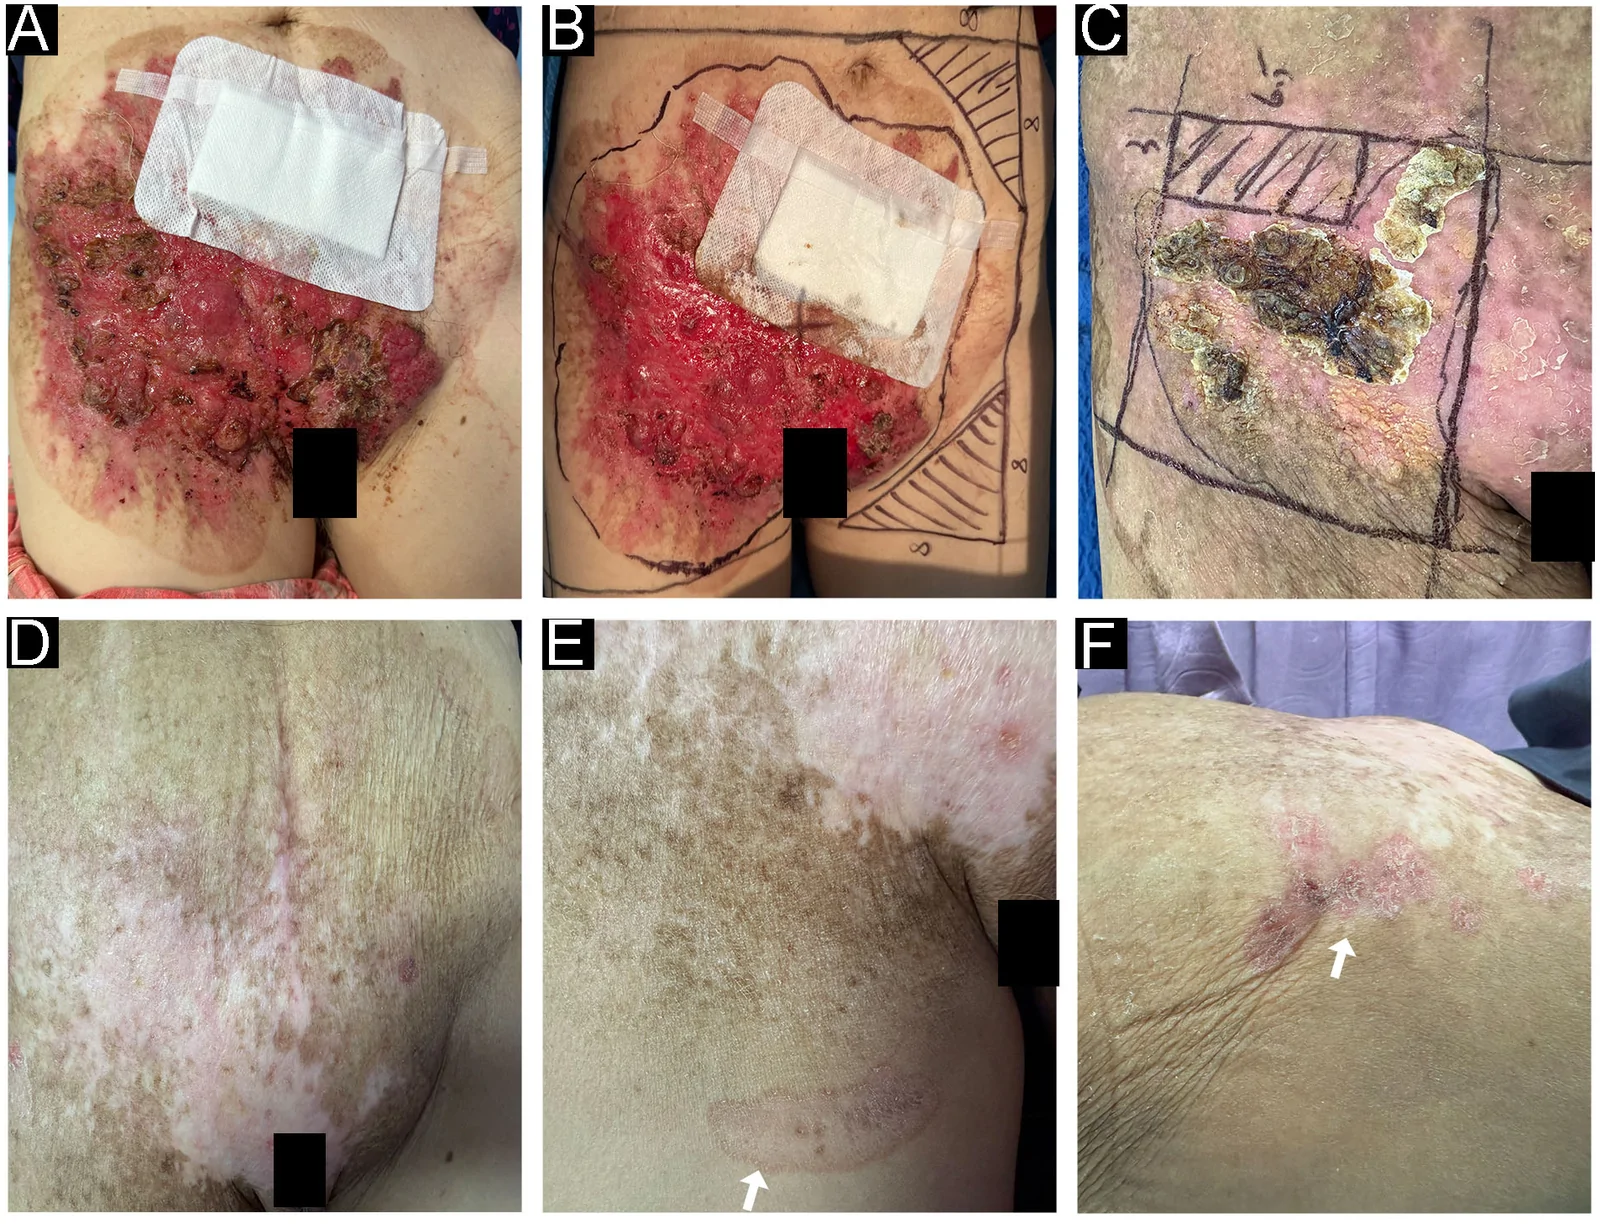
Clinical course and electron beam radiotherapy field design. (A) Pretreatment clinical photograph showing a large irregular erythematous and erosive plaque involving the lower abdominal-pelvic skin. (B) Skin surface markings for the initial electron field. The marked lines indicate the planned lead-shielding boundary and field-edge design rather than a formally contoured three-dimensional target volume; a 5-mm tissue-equivalent bolus was used to improve surface-dose coverage. (C) Marked regression after the initial 43.2-Gy course. The outlined area indicates the second-stage boost field, which was defined according to residual erythema, erosion, crusting, or thickening, with interim MRI used as supporting information. (D) Central lower abdominal-pelvic skin within the treated field at nearly 1 year after radiotherapy, showing complete clinical resolution with post-inflammatory pigmentation changes. (E) Peripheral lower abdominal-pelvic skin near the initial field edge showing a residual erythematous scaly plaque. (F) Adjacent inguinal region showing another residual out-of-field plaque, indicated by the white arrow.
